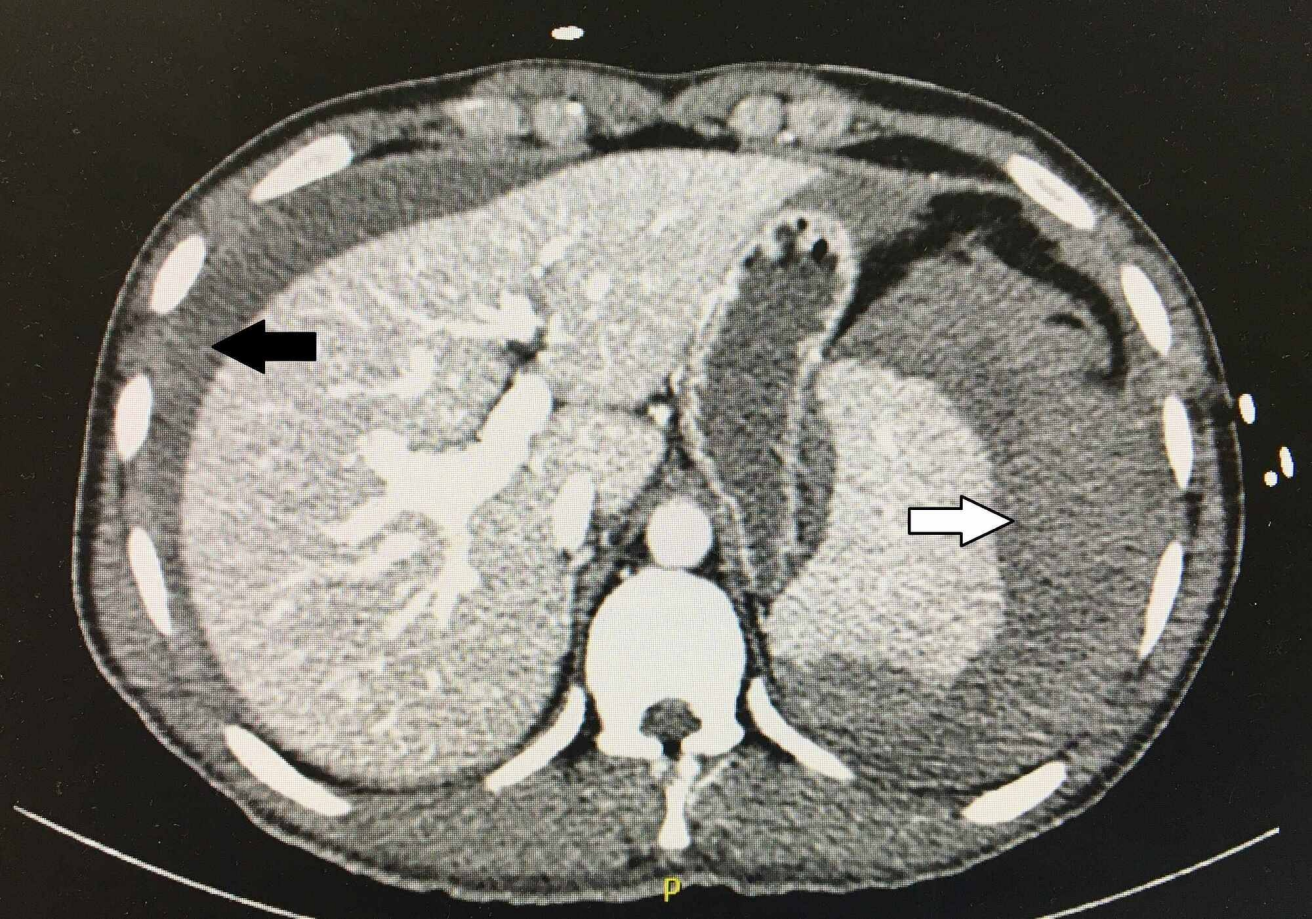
CT of the abdomen showing perisplenic hematoma (white arrow) and hemoperitoneum (black arrow).
